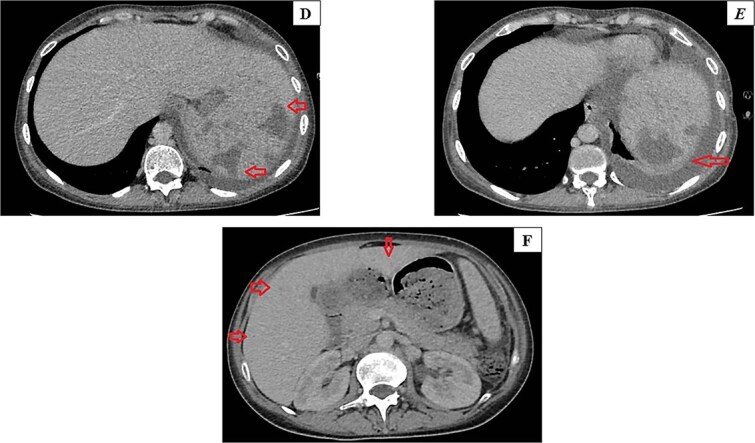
(D-F): Computed tomography (CT) scan showing hypodense lesions in spleen and liver.
